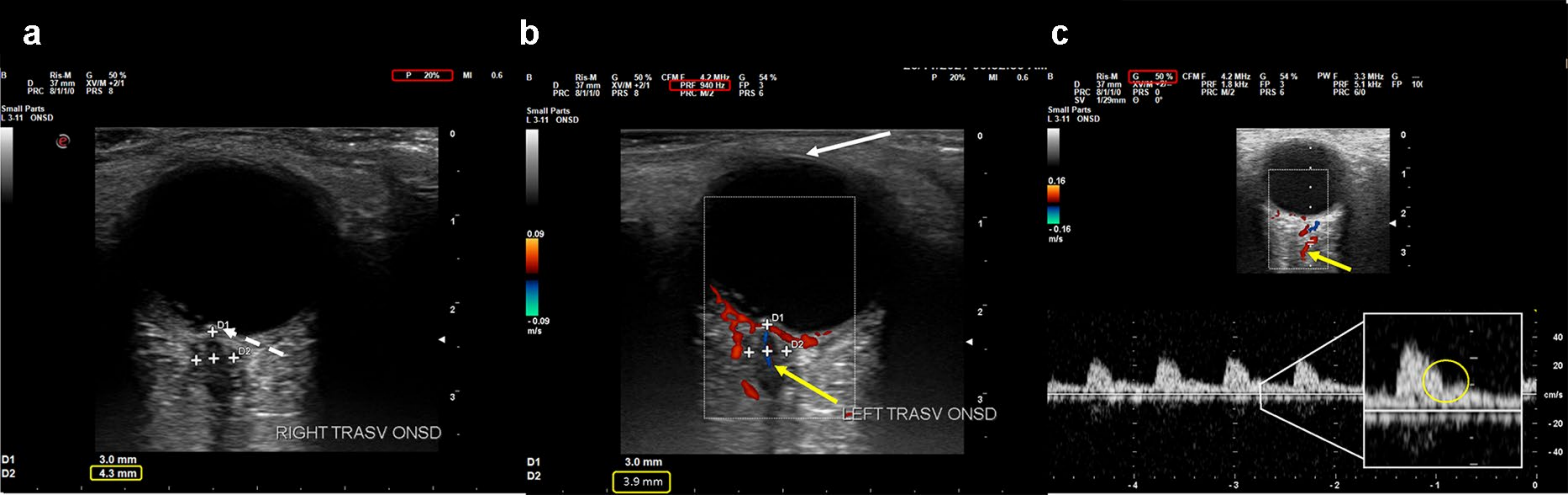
Three ultrasound images of the eyeball and optic nerve with sheath, enriched by the parameters/landmarks highlighted in CLOSED protocol. a B-mode image in gray-scale: dashed white arrow points to the optic disc, in yellow window 4.3 mm ONSD measurement, in red window acoustic power output = 20%; b same image but with color-mode: white arrow points to the lens, in yellow window 3.9 mm ONSD measurement, in red window PRF 940 Hz (almost 1 Kh), yellow arrow points to central retinal artery; c same image but with color-mode plus Doppler wave (at the bottom): in red window Gain 50%, yellow arrow points to ophthalmic artery, in the enlarged panel the typical nock of the ophthalmic artery flow
